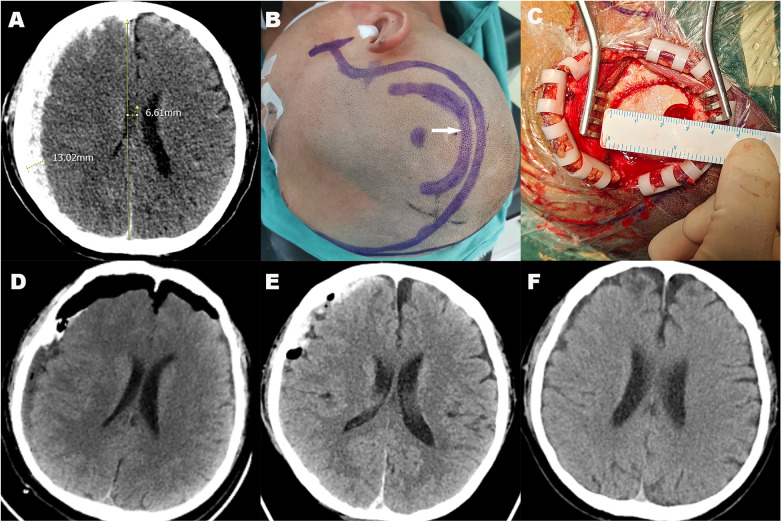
(A) Head CT scan showing a thick acute right-sided subdural hematoma and remarkable midline shift at the time of admission. (B) Curvilinear incision (white arrow) could facilitate conversion to a possible craniotomy. (C) The measured size of the bone flap was 3 cm. (D–F) Follow-up CT on immediate postoperation, 4, and 25 days later, respectively.
